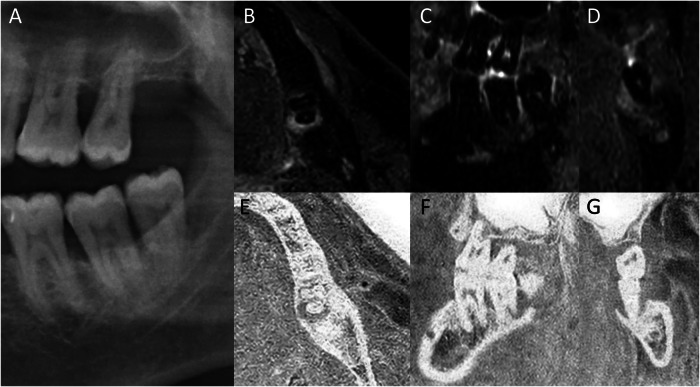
63-year-old male patient with a periapical lesion of the distal root of tooth 37. No periapical radiolucency (thick white arrow) is visible in OPT (A). The axial (B), sagittal (C) and coronal (D) reconstructions of a 3D STIR sequence show a large bone marrow edema around a periapical lesion, with a volume of 127 mm^3^ and a signal-to-noise ratio of 2,16. The axial (E), sagittal (F) and coronal (G) reconstructions of a 3D T1 sequence show a corresponding small bone defect with a volume of 65 mm^3^
